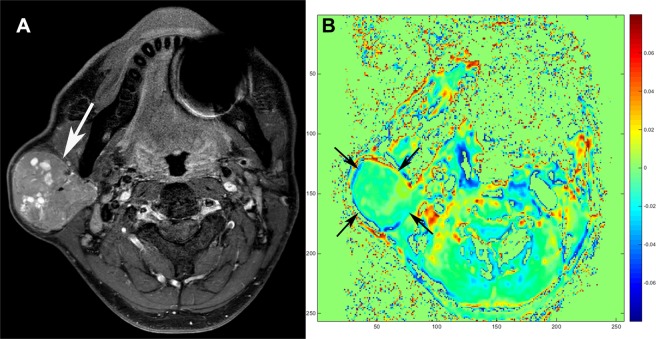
Warthin tumor of a 46-year-old male. (A) Axial T2-weighted imaging with fat suppression shows a well-defined hyperintense mass with multifocal cystic portion in the right parotid gland (arrow). (B) Amide proton transfer-weighted (APTw)-MRI demonstrates that APTw-signal of the tumor is relatively low (arrows). The averaged APTw-signal values obtained by the two readers in the solid portion were as follows: Mean APTw-signal, −2.36%; maximum APTw-signal, −0.53%; median APTw-signal, −2.35%.
